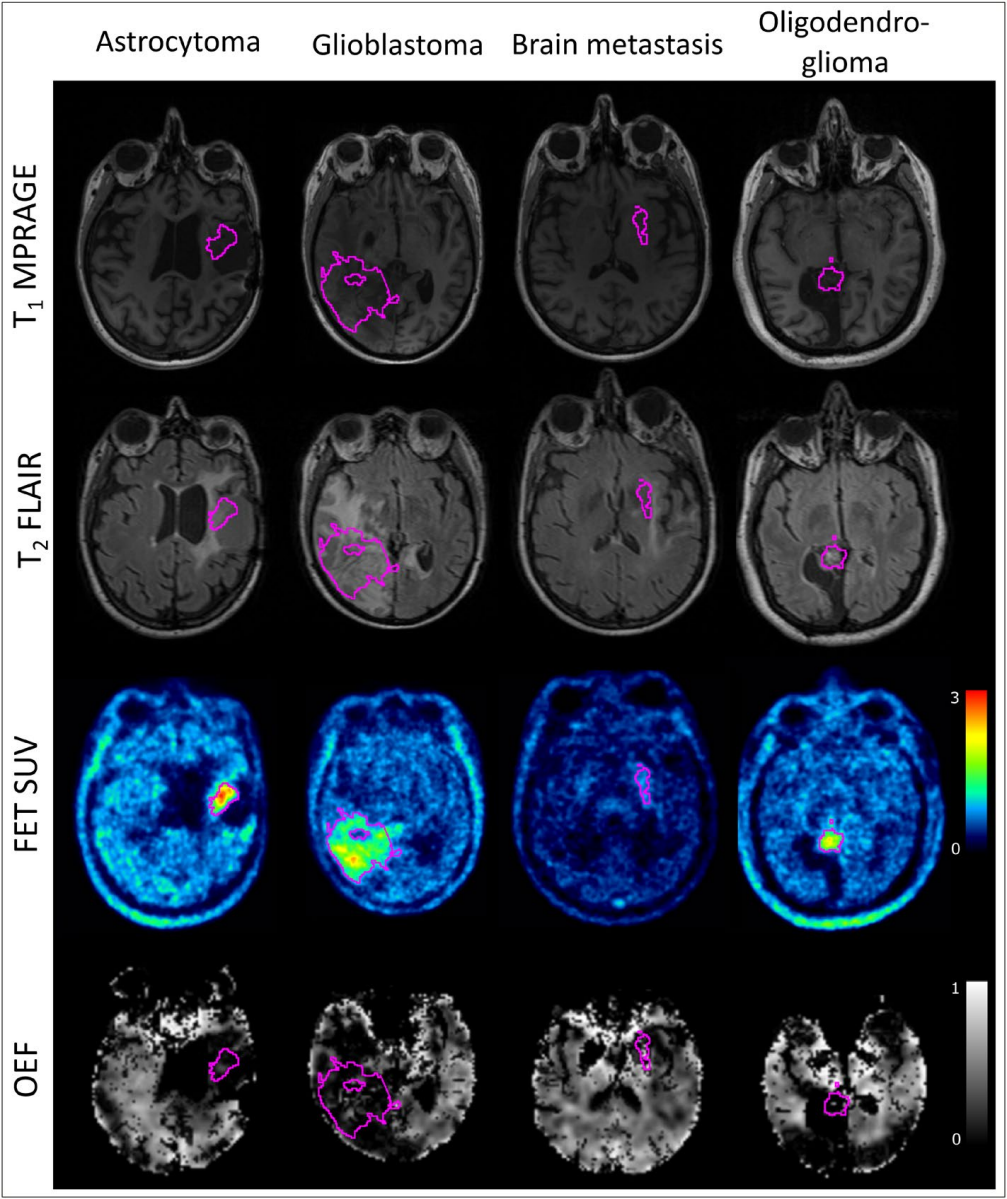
Representative images of the anatomical T1 MP‐RAGE scan (top), T2w FLAIR images (2nd row), the FET SUV map (3rd row) and OEF maps (bottom) for different tumor types, that is, astrocytoma, glioblastoma, metastasis, and oligodendroglioma, from left to right. Tumor VOIs derived from FET PET thresholds are overlayed with pink outlines.
